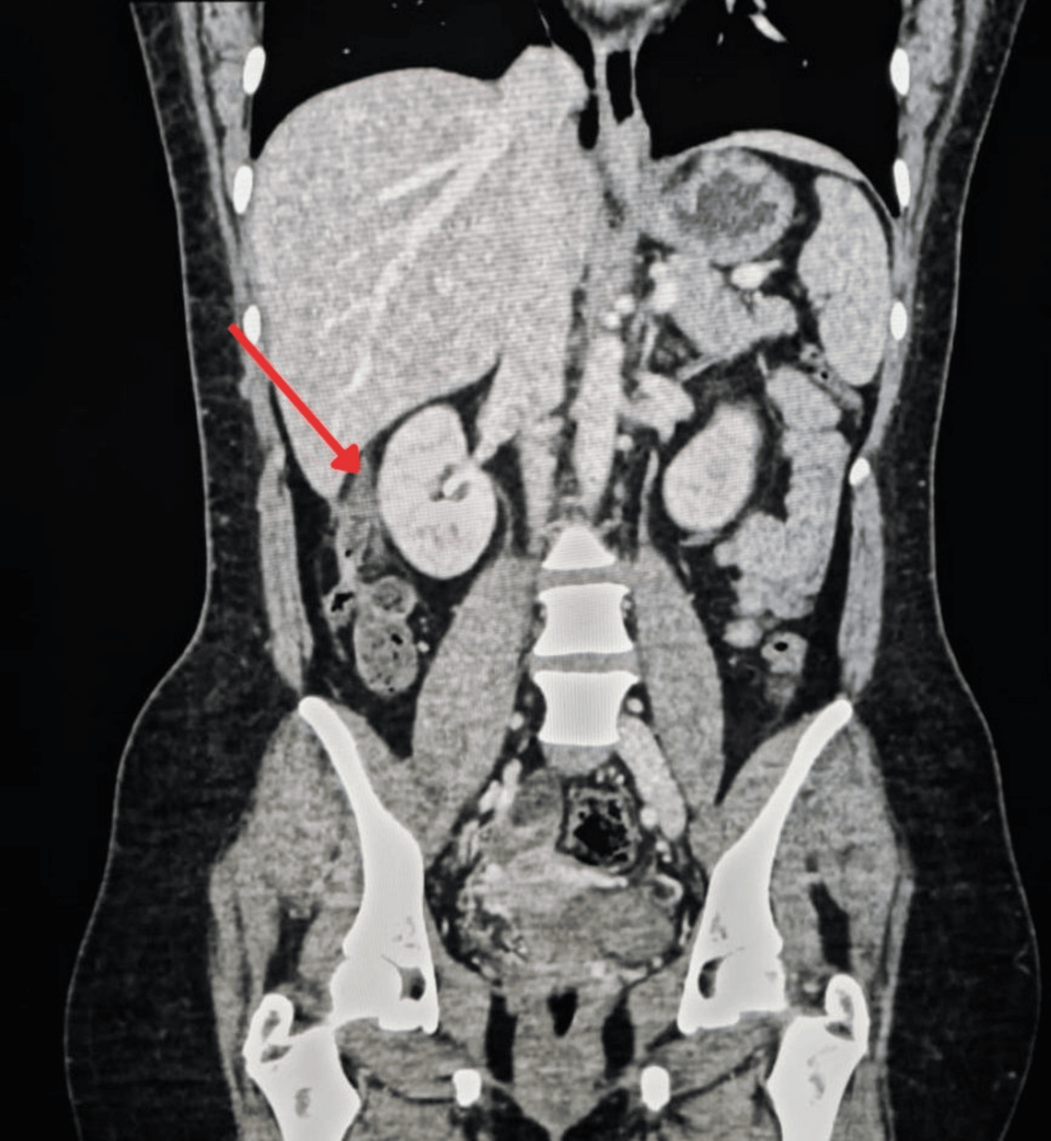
Computed tomographic image showing subhepatic appendicitis (arrow)
